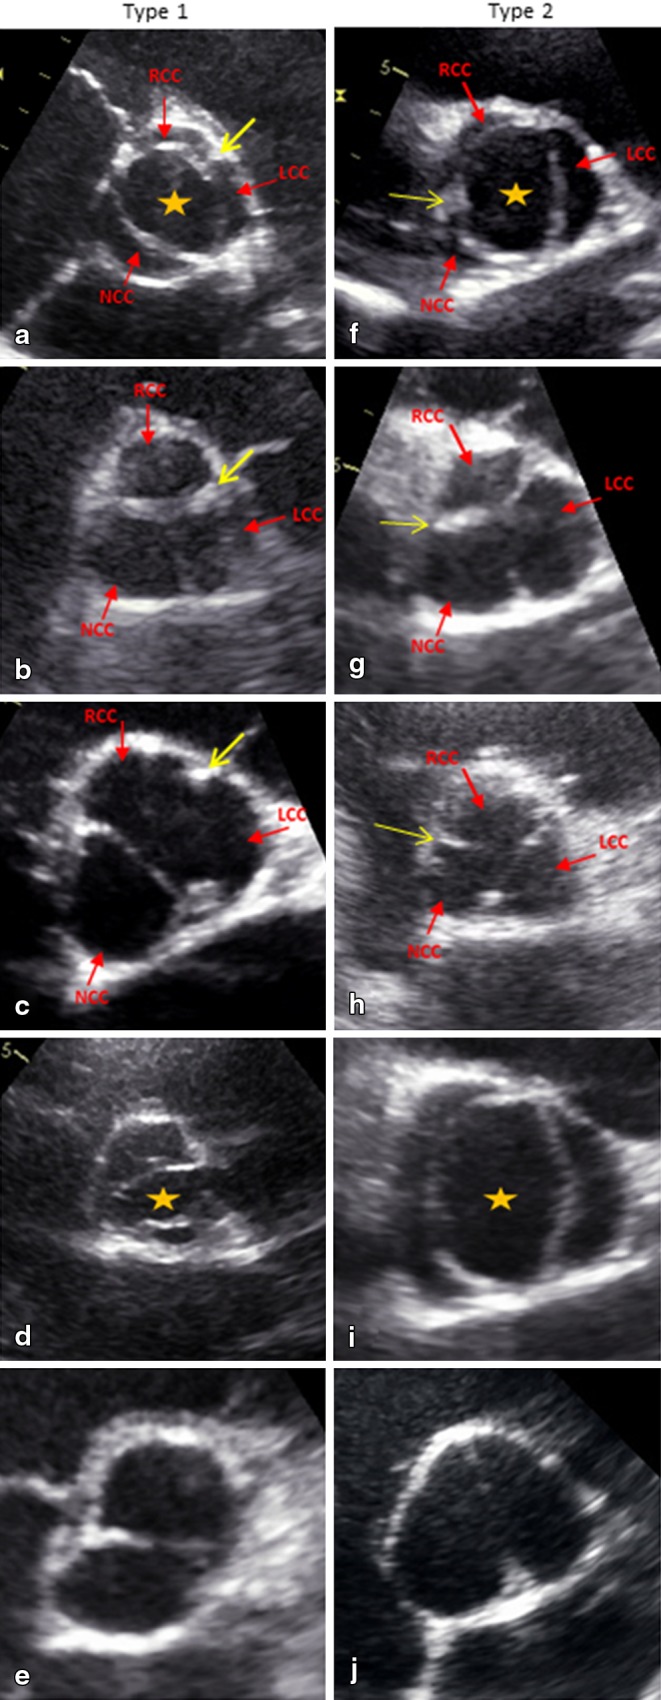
Representative examples of different bicuspid aortic valve (BAV) types as seen from the short-axis parasternal view on echocardiography. The *yellow arrow* indicates the raphe. The orange star indicates the typical fish mouth opening of the valve. a Type 1A in open position. b Type 1A with complete raphe in closed position. c Type 1A with incomplete raphe in closed position. d Type 1B in open position. e Type 1B in closed position. f Type 2A in open position. g Type 2A with complete raphe in closed position. h Type 2A with incomplete raphe in closed position. i Type 2B in open position. j Type 2B in closed position
